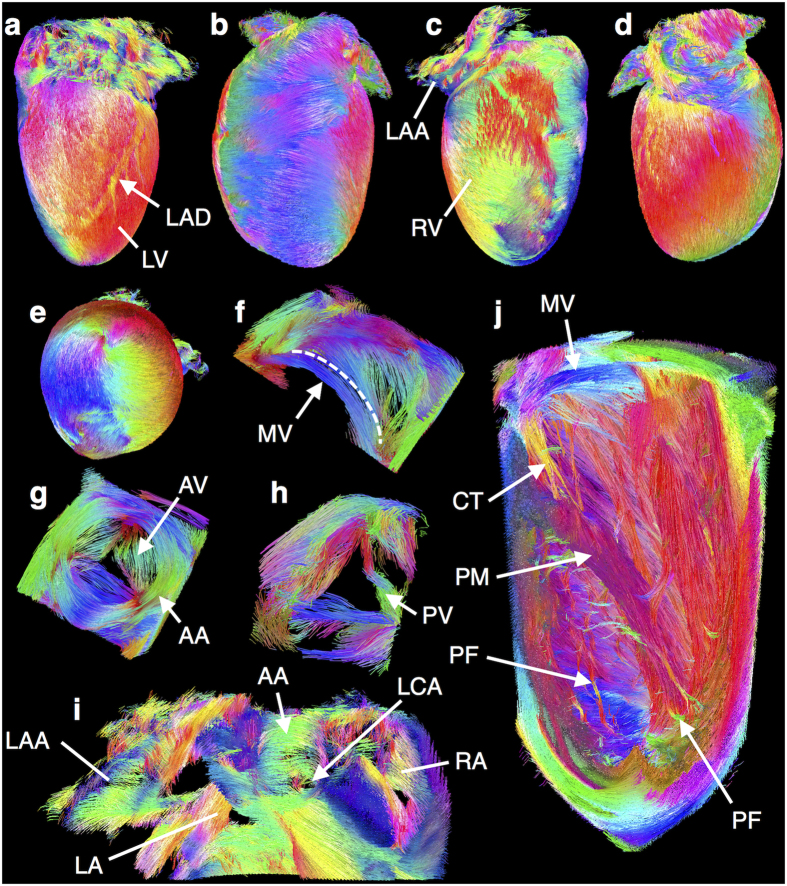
Eigenvector tracking in the whole heart. Tracks were reconstructed based on v_1_ and colour-coded by orientation: apico-basal (red), anterior-posterior (green) and lateral-septal (blue). Tracks in the heart from (a) lateral, (b) anterior, (c) septal, (d) posterior and (e) apical views. Left helical tracks of the LV subepicardium and circumferential tracks of the RV myocardium are seen. Gaps in subepicardial tracks correspond to the left anterior descending artery (LAD). Tracks can be seen to spiral towards the apex. (f) Tracks in the leaflets of the mitral valve (MV) are primarily oriented parallel to the boundary between leaflets (dashed line). (g) Tracks in the vessel wall of the aorta (AA) are aligned primarily in a circumferential direction, while in the cusps of the aortic valve (AV) they are oriented in an equilateral configuration before curving basally into their insertions into the aortic wall. (h) The pulmonary valve (PV) comprises three leaflets, with tracks organised in an equilateral configuration. (i) Tracks in the left and right atria (LA and RA), and left atrial appendage (LAA) viewed from the septal wall. The left coronary artery (LCA) is seen extending from the aorta and tracks in its vessel walls are aligned circumferentially. (j) Tracks in a 13 × 5 × 5 mm section in the LV reveal chordae tendinae (CT), oriented longitudinally between their connections to the mitral valve and papillary muscle (PM). Networks of Purkinje fibres (PF) can be seen towards the apex.
